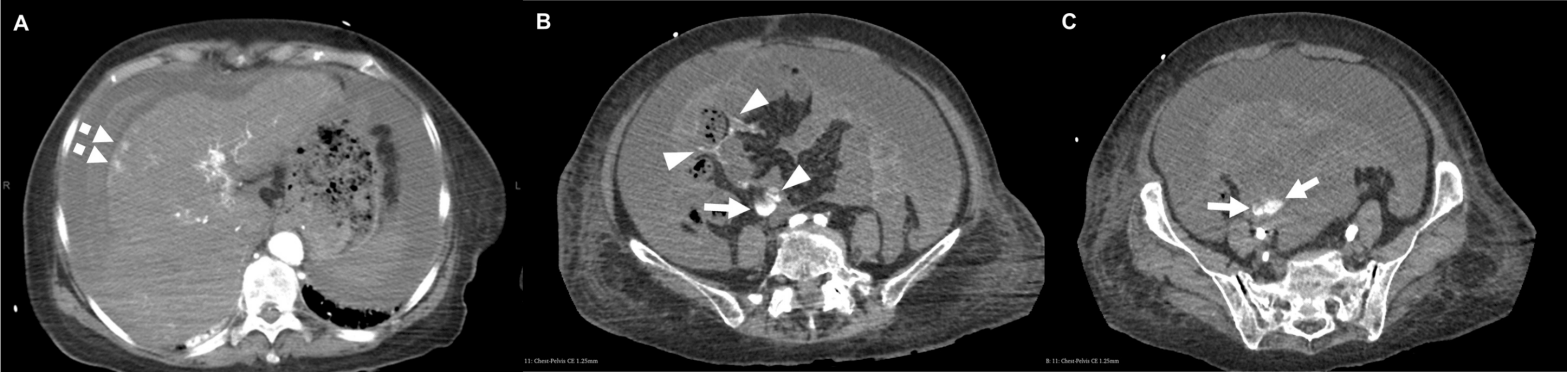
Abdominal contrast-enhanced CT scan showing intraperitoneal hemorrhage from ectopic varices rupture (A) A dense hematoma around the liver and parenchymal enhancements (dotted arrows), suggestive of infiltrative hepatocellular carcinoma. (B and C) An ectopic varix of the superior mesenteric vein (arrows) and contrast extravasation (arrowheads).
